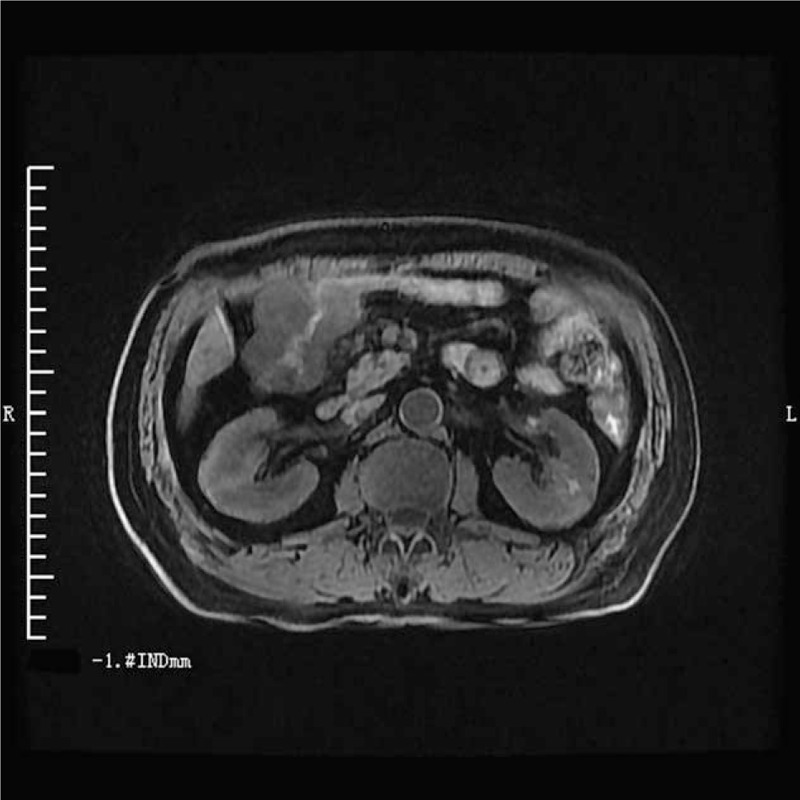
Imaging findings. Abdominal MRI showed an irregular thickening of the hepatic flexure of colon with narrow enteric cavity.
